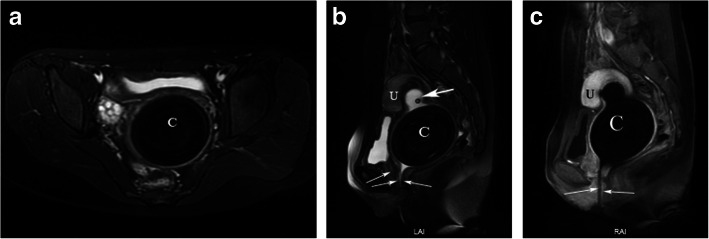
a Axial and b Sagittal T2-weighted imaging with fat saturation of pelvis, showing a large, extremely low-signal intensity mass (c) in the vagina that caused the uterus moved upward. A balloon catheter shadow (thick arrow) can be seen above the mass. The urethra and vagina form a common channel (arrow). c Sagittal contrast-enhancement T1-weighted imaging with fat saturation of pelvis, showing the mass has no enhancement
